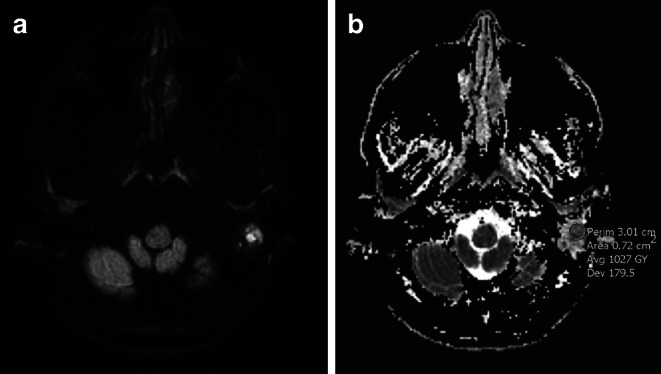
Restricted intramastoid diffusion due to purulent infection on axial DWI trace map (a) and ADC map (b) of the skull base. Note that mastoid may simultaneously include areas with different diffusion restriction. Measurement of ADC values was performed from areas with the most restriction, such as on b
